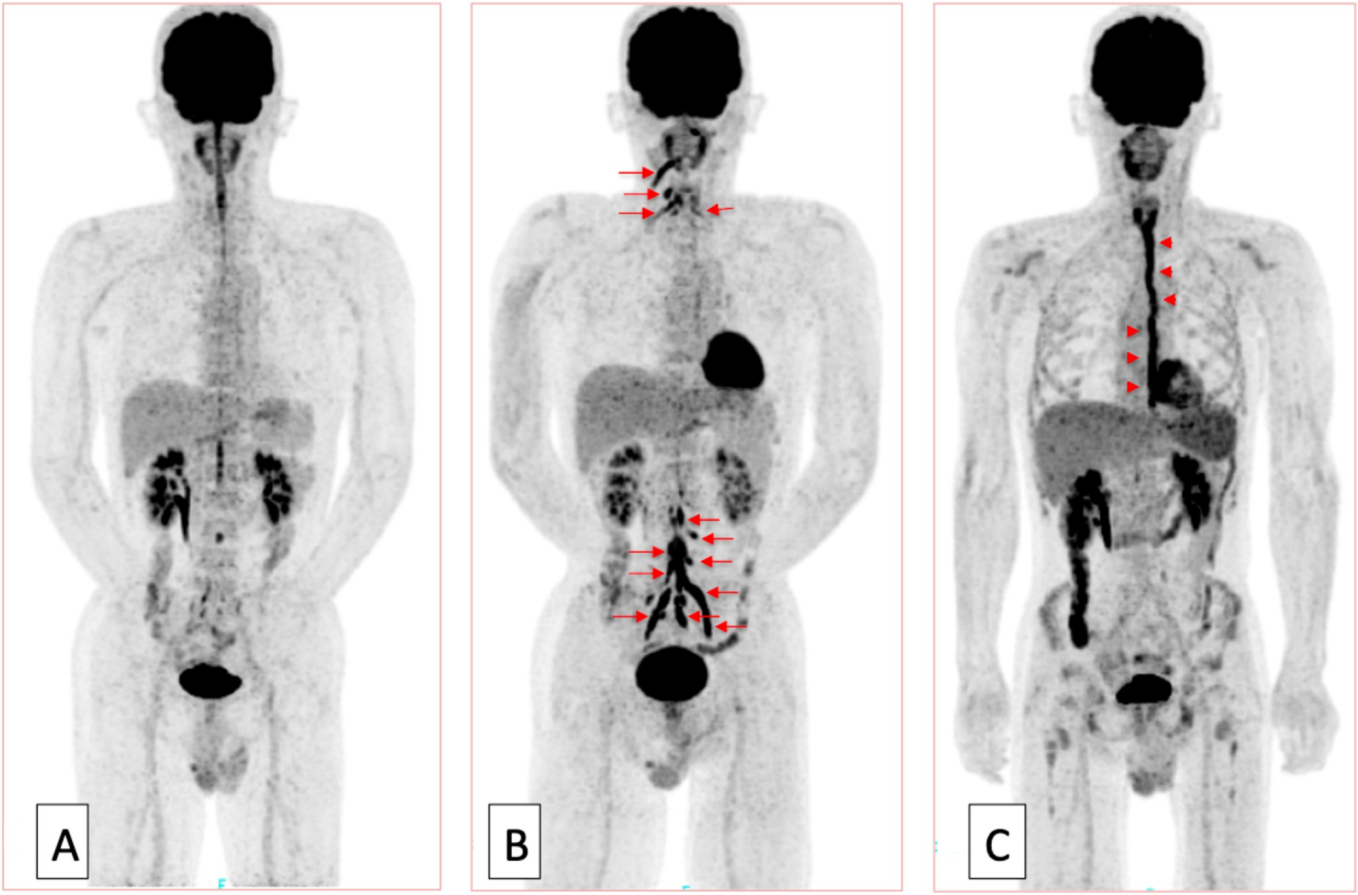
Fluorodeoxyglucose positron emission tomography (FDG-PET) at presentation, after chemotherapy, and after craniospinal irradiation FDG-PET at presentation (A), following chemotherapy with high-dose methotrexate (MTX) (B), and after craniospinal irradiation (C). FDG-PET after high-dose MTX showed accumulation of FDG in the nerve roots somewhat extending somewhat further into the peripheral portions (B, orange arrows), which had not been noted before previously (A). In addition, this high-uptake area almost completely disappeared after conventional radiation therapy (C).  Accumulation of FDG along the esophagus was due to radiation esophagitis (C, orange arrowheads).
